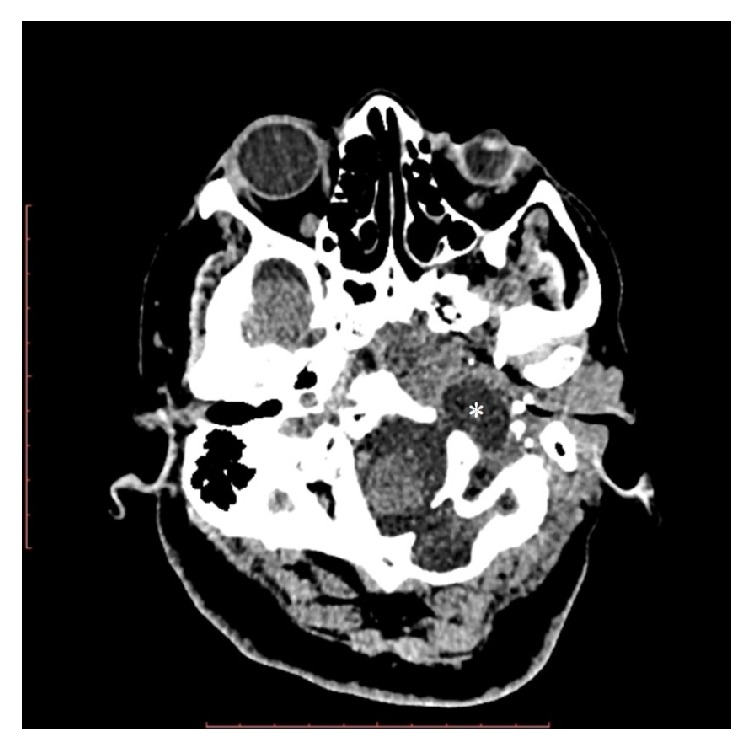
Axial CT image with bone window settings shows enlargement of left jugular foramen (*∗*).
